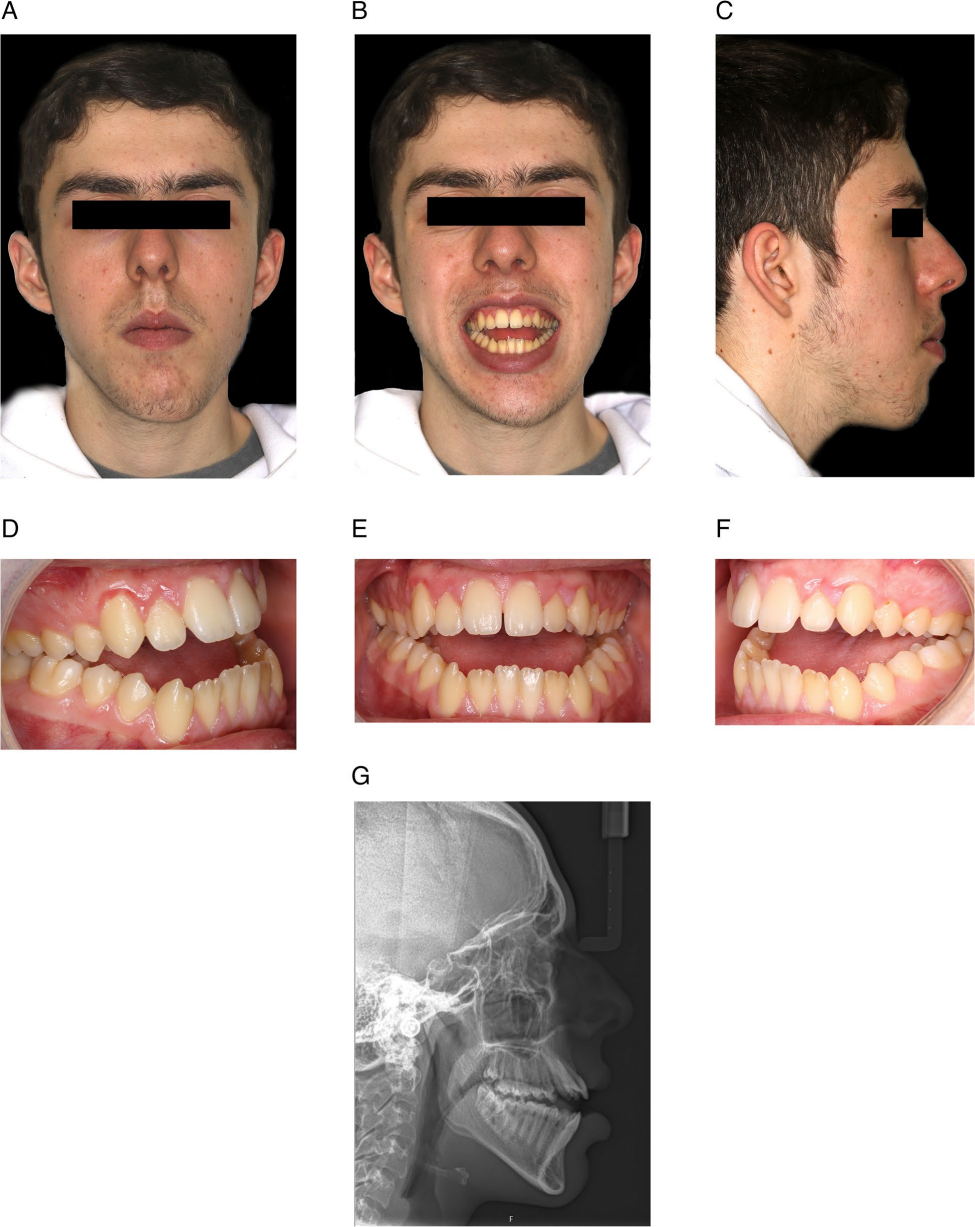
Pretreatment clinical photographs and lateral cephalometric radiograph
